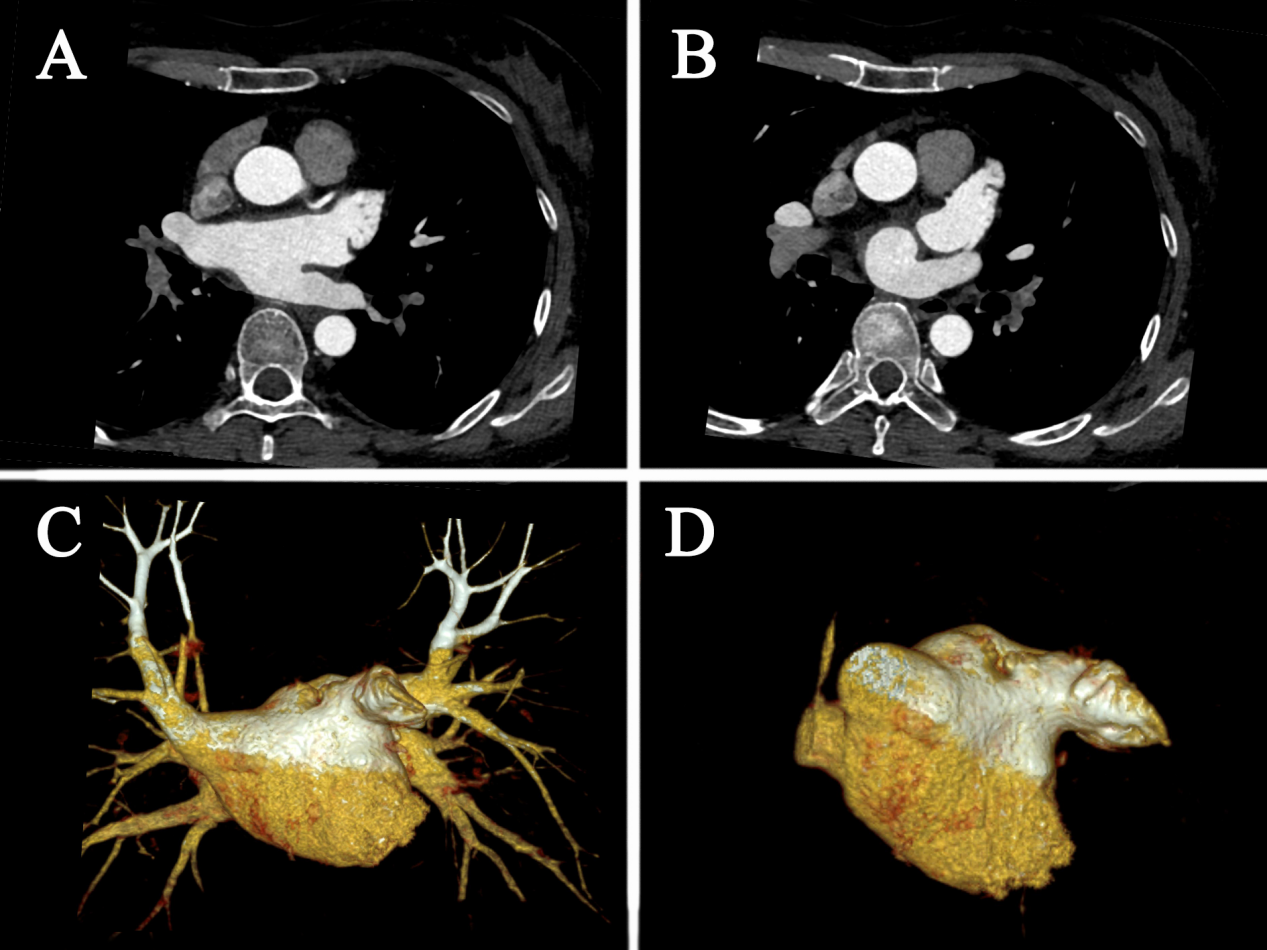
Cardiac CT images. (A) axis position; (B) axis position; (C)
